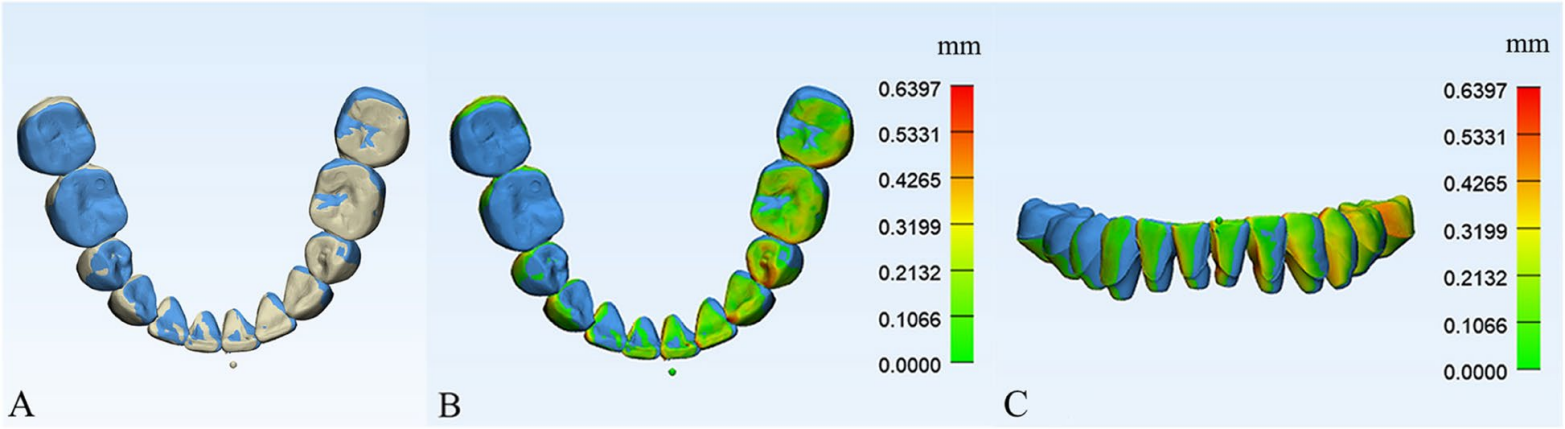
Part comparison analysis. A Top view of DM-CBCT-T2 (blue) and DM-V-T2 (yellow). B, C Top view and frontal view of part comparison analysis of two parts, the color bar (range from green to red) shows the differences
